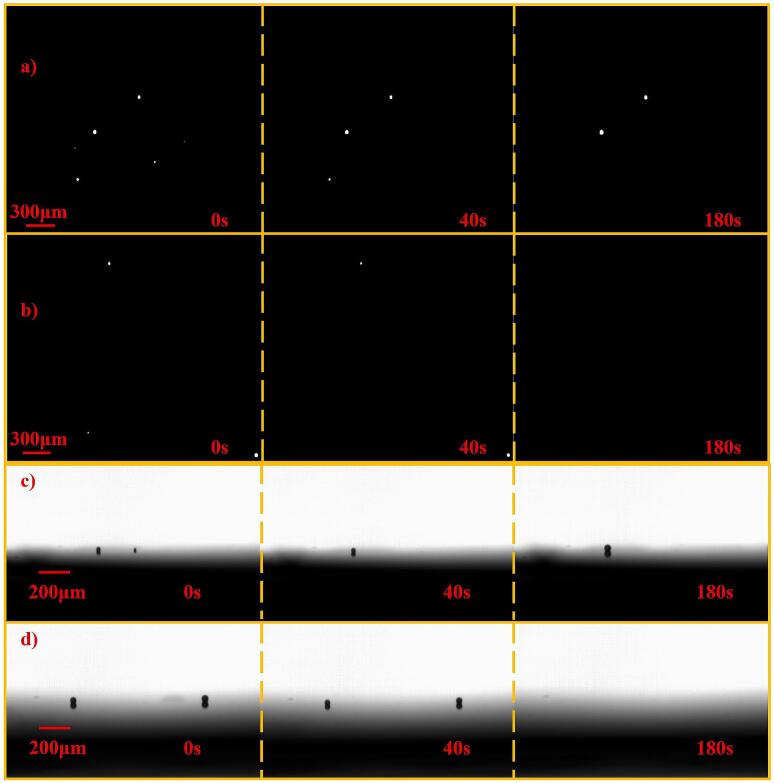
Top-view images of surface microbubbles on a hydrophilic surface in OW (a) and SW (b), and side-view images in OW (c) and SW (d), acquired at 0 s, 40 s, and 180 s after ultrasonication.
